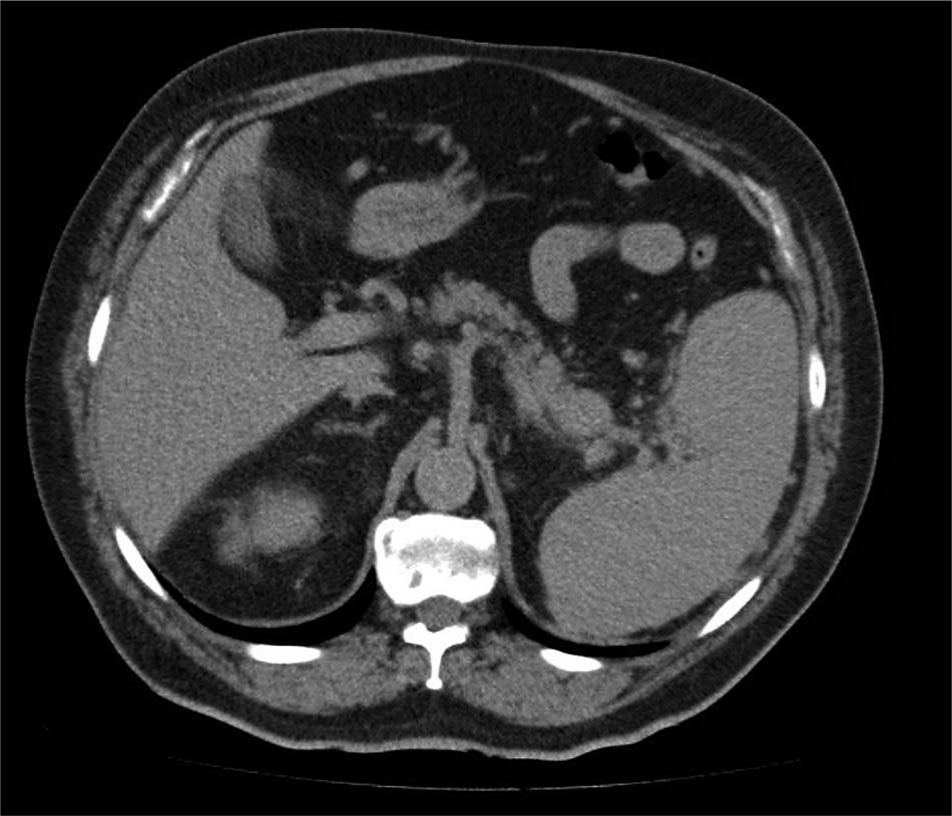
A contrast-enhanced CT scan of the abdomen (axial image) showing a soft tissue lesion in the pancreatic tail measuring 2.4 × 2.7 cm, radiologically suspicious for a primary pancreatic neoplasm.
